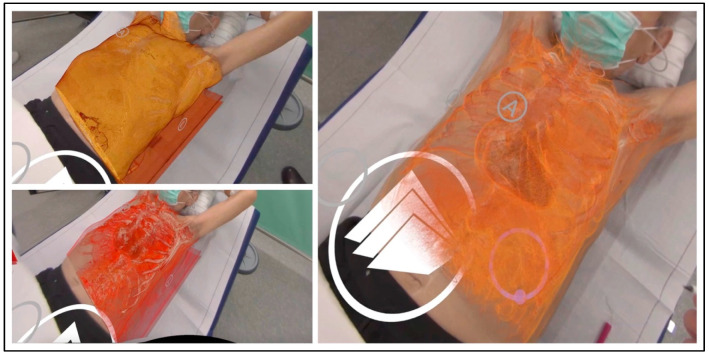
Alignment and windowing of high-resolution reconstructed image on patient with large epithelioid sarcoma. The anterior side of the rendered CT image is labeled Ⓐ.
